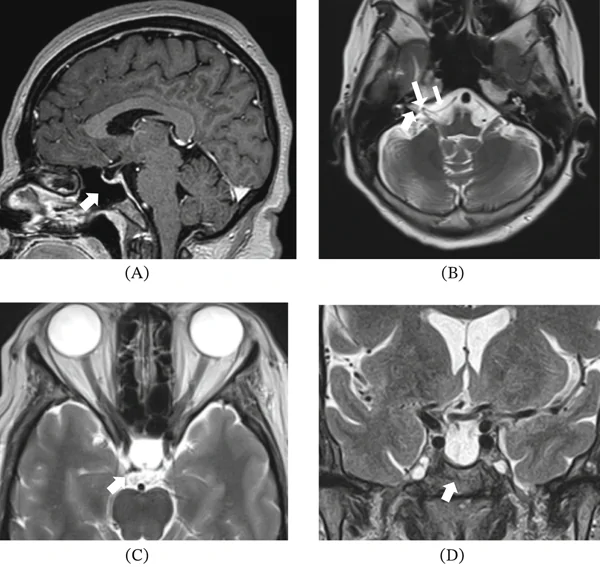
(A) Contrast‐enhanced brain MRI demonstrating a Grade IV sellar arachnoidocele, consistent with an empty sella configuration. (B) T2‐weighted MRI of the cerebellopontine angle showing a right‐sided vascular loop (Chavda Grade I) in close contact with the facial (CN VII) and vestibulocochlear (CN VIII) nerves. The nerves and the adjacent vascular loop are marked with arrows (CN VII, arrowhead; CN VIII, thin arrow; vascular loop, thick arrow). (C) Sagittal T2‐weighted brain MRI demonstrating an empty sella turcica, characterized by CSF filling the sellar cavity with flattening of the pituitary gland. (D) Coronal T2‐weighted brain MRI showing the empty sella with CSF occupying the sella.
